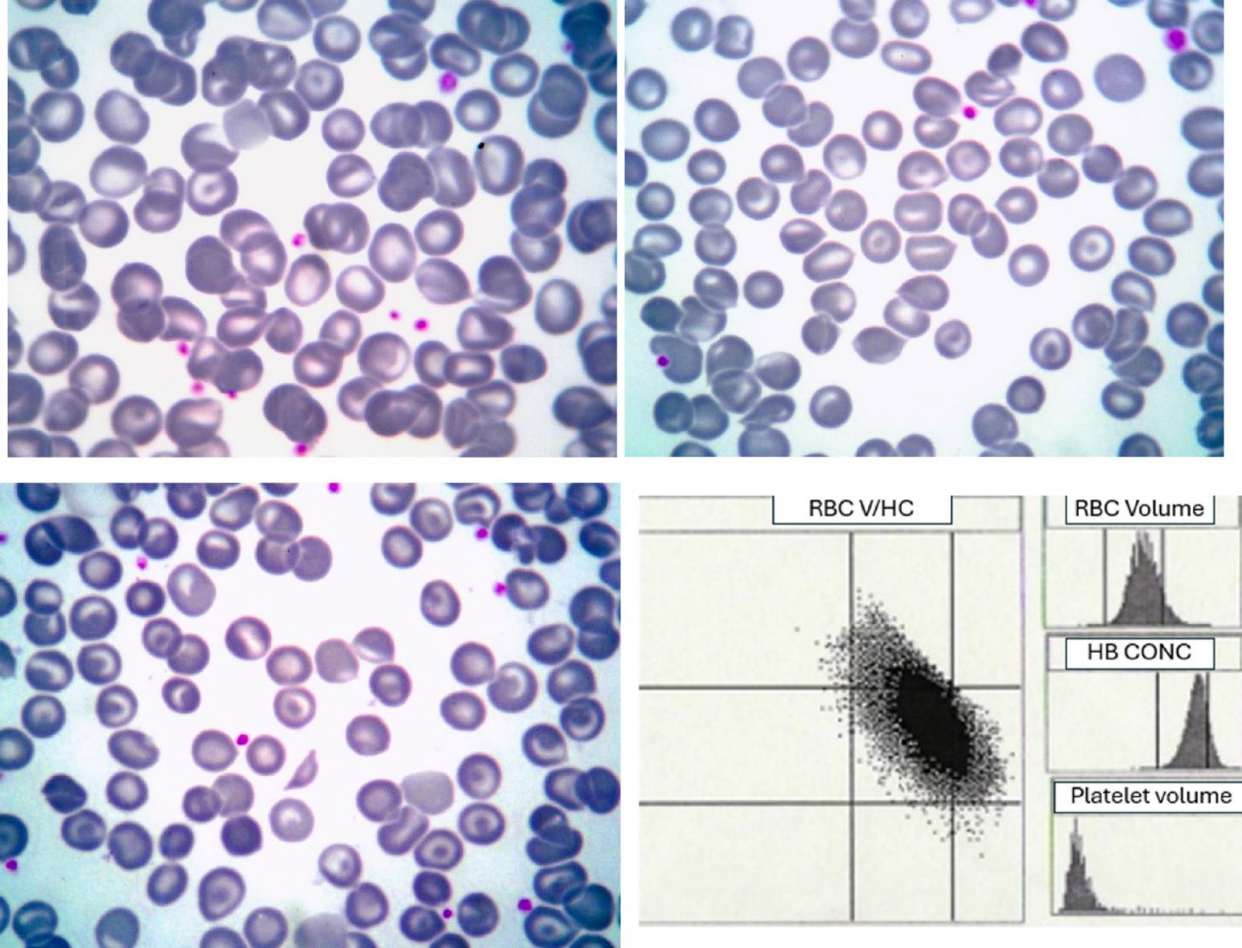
A male patient, now 67‐years‐old, was referred in 1997 for evaluation of suspected hereditary hemochromatosis and for initiation of regular phlebotomy. His blood count showed hemoglobin concentration (Hb) 166 g/L, mean corpuscular volume (MCV) 112.7 fL, mean corpuscular hemoglobin (MCH) 37.8 pg, and mean corpuscular hemoglobin concentration (MCHC) 33.6 g/dL. Platelet and leukocyte counts were normal. Serum iron was 154 μg/dL, transferrin 170 mg/dL, transferrin saturation 90.7%, and ferritin 956.5 ng/mL. Total bilirubin was 1.82 mg/dL with a direct fraction of 0.51 mg/dL. Haptoglobin was 79 mg/dL. Abdominal ultrasonography showed hepatomegaly with a homogeneous liver texture, which was confirmed by computed tomography showing mild parenchymal hyperdensity, likely reflecting increased hepatic iron deposition. Liver biopsy demonstrated grade IV/IV hemosiderosis and septal fibrosis. Based on these findings, a diagnosis of hereditary hemochromatosis and Gilbert syndrome was favored, and regular phlebotomy was commenced. However, genetic testing for common *HFE* mutations (H63D, C282Y, and S65C) was negative, and careful examination of a blood smear (upper images and lower left, May‐Grünwald‐Giemsa, ×100 objective) revealed stomatocytes, knizocytes (stomatocytes with two indentations), irregularly contracted cells, spherocytes, target cells, and occasional schistocytes. The reticulocyte count was consistently elevated (~230 × 10^9^/L). Osmotic fragility testing showed increased red cell resistance. Red cell cytograms showed a marked increase in cells with an increased hemoglobin concentration (lower right image, Advia). Hemoglobin electrophoresis and assays for glucose‐6‐phosphate dehydrogenase and pyruvate kinase were normal.
